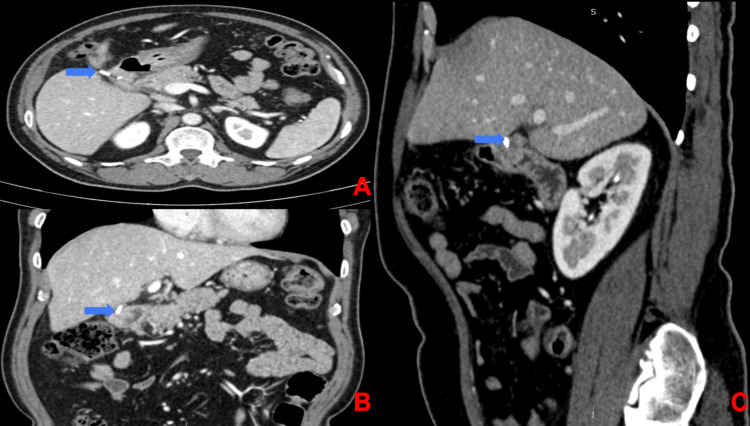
Contrast-enhanced computed tomography (A: transverse section, B: coronal section, C: sagittal section) of the abdomen showed an impacted surgical clip (blue arrow) into the lateral wall of the first part of the duodenum with nodular soft tissue adjacent to it and minimal pyloroduodenal thickening.
